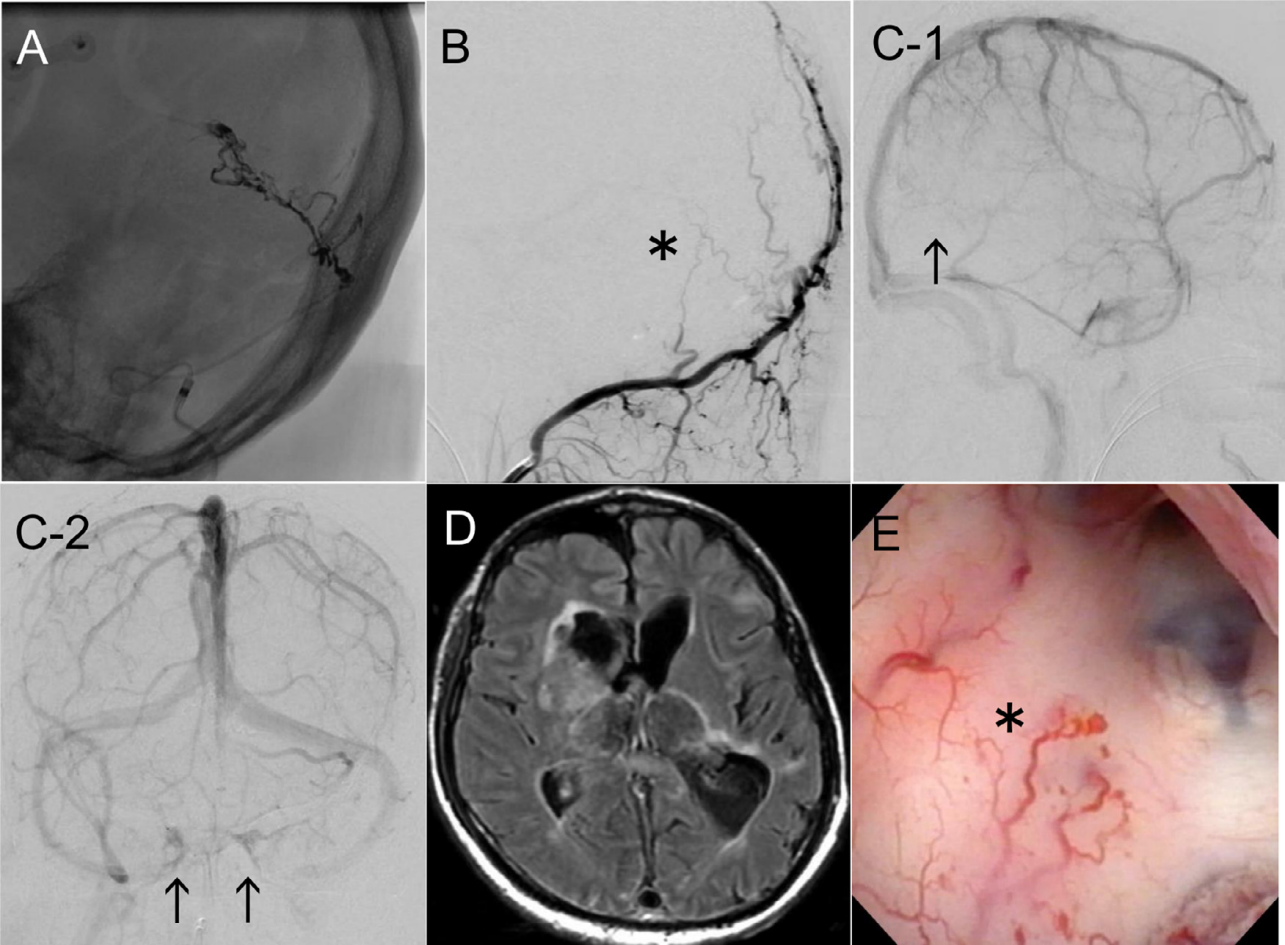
(A) TAE was performed for the shunt point occlusion. Injection from Scepter XC cannulated to the left OA shows shunt flow from the mastoid branch to the StS. Onyx 18 was injected intermittently with balloon inflation, a total of 1.6 mL in 10 minutes. (B) Arteriovenous shunt occlusion was confirmed on postembolization left OA angiography (asterisk, lateral view). (C) Postembolization right ICAG showing remnant of StS occlusion (C-1, arrow), and patency of CS as venous drainage route (C-2, arrow). (D) MRI FLAIR showing bilateral thalamus injury due to high venous pressure by StS DAVF. (E) Intraoperative neuroendoscopy showing hypervascularity on the ventricular wall (asterisk), suggesting congestion of venous return or the effect of angiogenesis. CS, cavernous sinus; DAVF, dural arteriovenous fistula; ICAG, internal carotid artery angiography; MRI FLAIR, magnetic resonance fluid-attenuated inversion recovery imaging; OA, occipital artery; StS, straight sinus; TAE, trans-arterial embolization.
